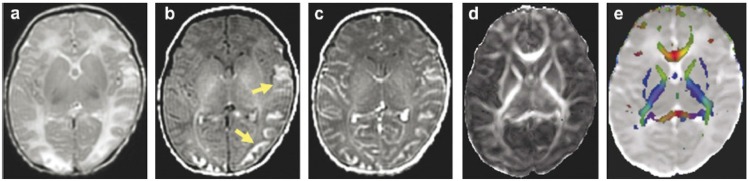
A case with clinical and para-clinical confirmed meningitis. There are signal alteration in the bilateral cortex, subcortex, and periventricular white matter of occipital area (arrow) and left temporal cortex on T2-weighted (a)/T1-weighted (b) images, respectively. The increased signal in cortex (b) is consistent with cortical laminar necrosis. Meningeal enhancement in gadolinium- T1-weighted (c). Reduced FA values in periventricular white matter in FA map (d). The abnormalities visible on T1 sequence are not demonstrated on FA map (d) and color coded FA (e) fused with MD (Malik et al., 2008).
